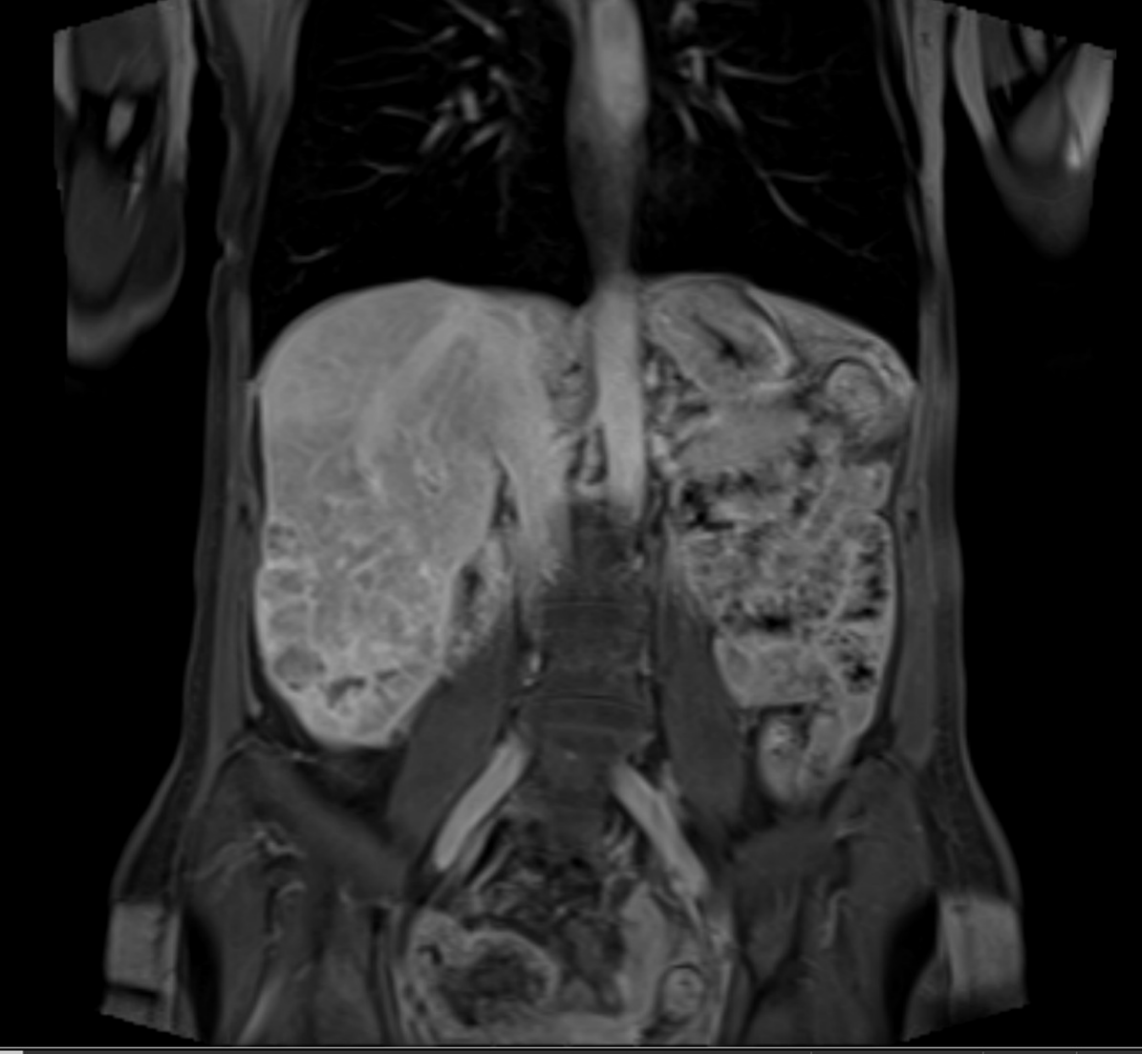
Representative coronal slice from MRI obtained after first SIRT procedure
